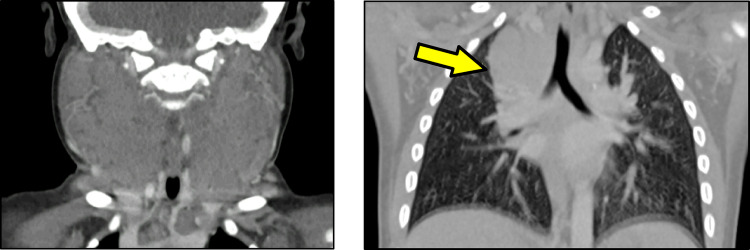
Contrast-enhanced CT images. The left image represents a coronal CT image of the neck demonstrating extensive bilateral cervical lymphadenopathy. The right image demonstrates a coronal CT image of the chest showing right mediastinal lymphadenopathy (yellow arrow) Immunophenotyping parameters of the present case
